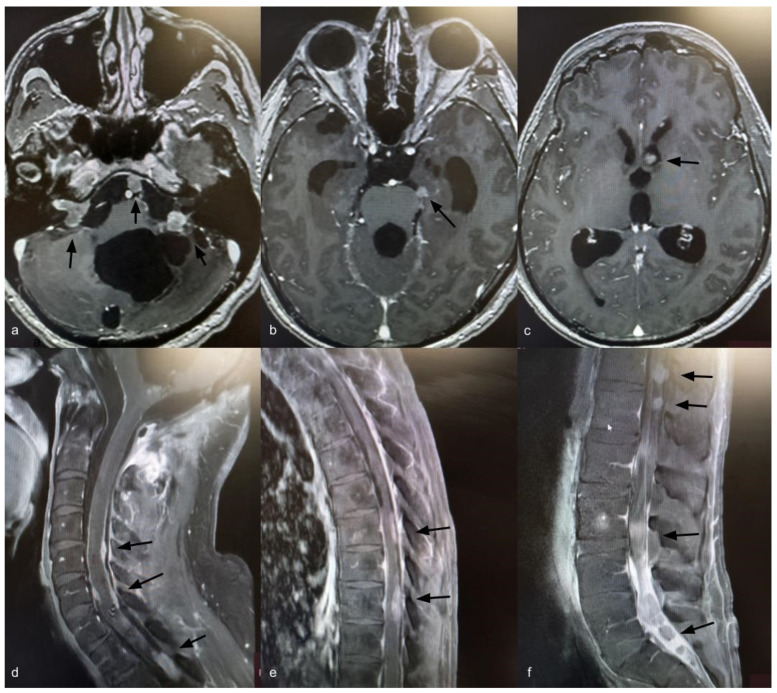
Axial T1-weighted brain MRI with gadolinium showing ventriculomegaly and multiple posterior fossa lesions at the right internal auditory canal, left cerebellopontine angle, prepontine cistern, and left frontal ventricular horn (a–c) (black arrows). Sagittal T1-weighted spine MRI with gadolinium showing leptomeningeal dissemination (d–f) (black arrows).
